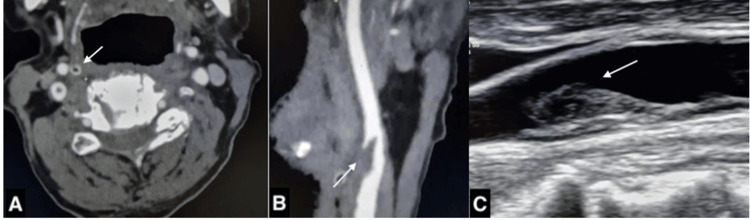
(A) The “donut sign” in a cross section (B) a free-floating thrombus attached to the carotid bulb and extending distally. (C) Carotid free-floating thrombus on a duplex ultrasound as a hyperechogenic formation arising proximally from the carotid wall and extending free to the arterial lumen distally
